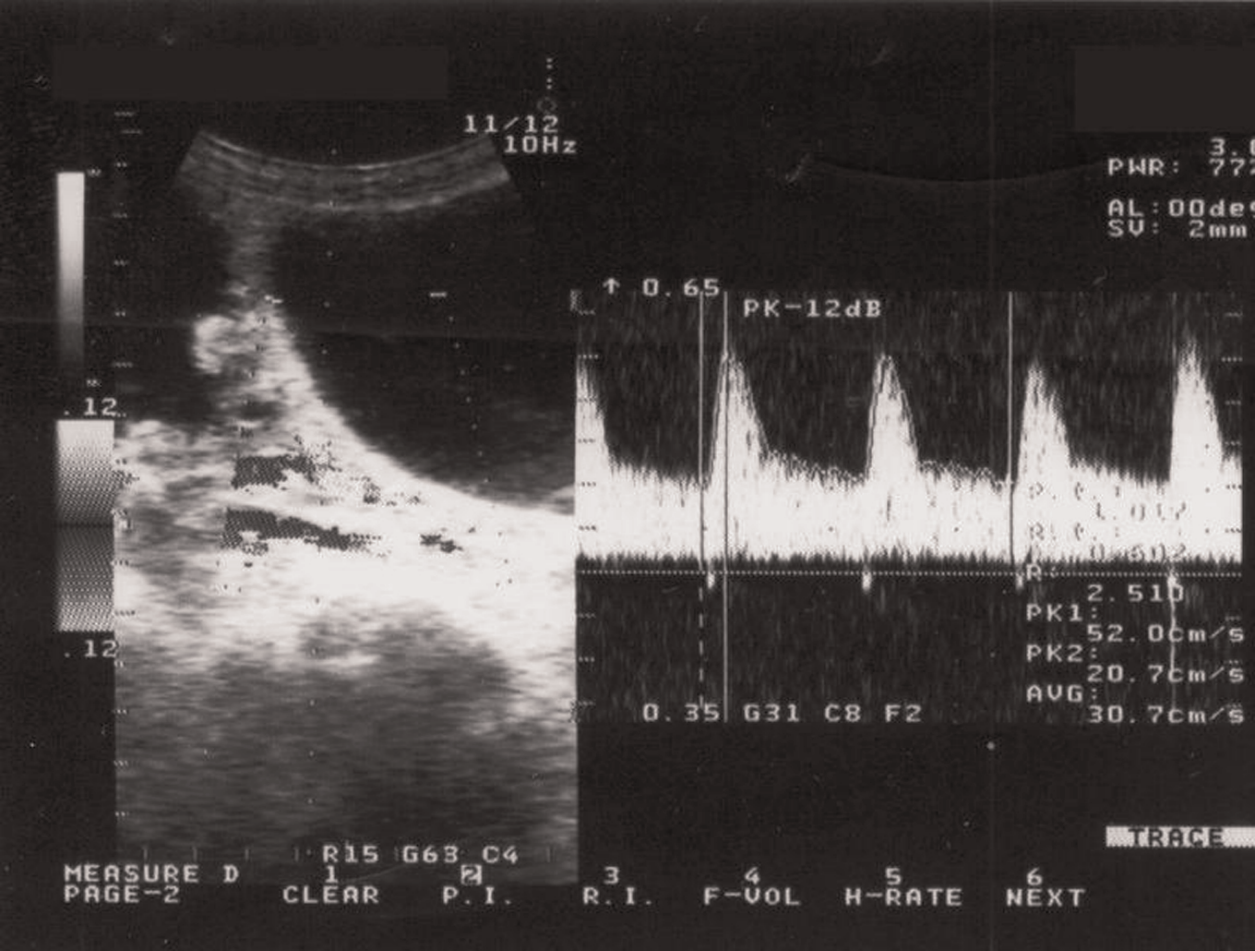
Transabdominal triplex sonogram of the vasculature of the right ovary after paracentesis of the giant cyst. The pulsatility index and the resistance index of the Doppler waveform of the vessels is 1.017 and 0.602 respectively.
